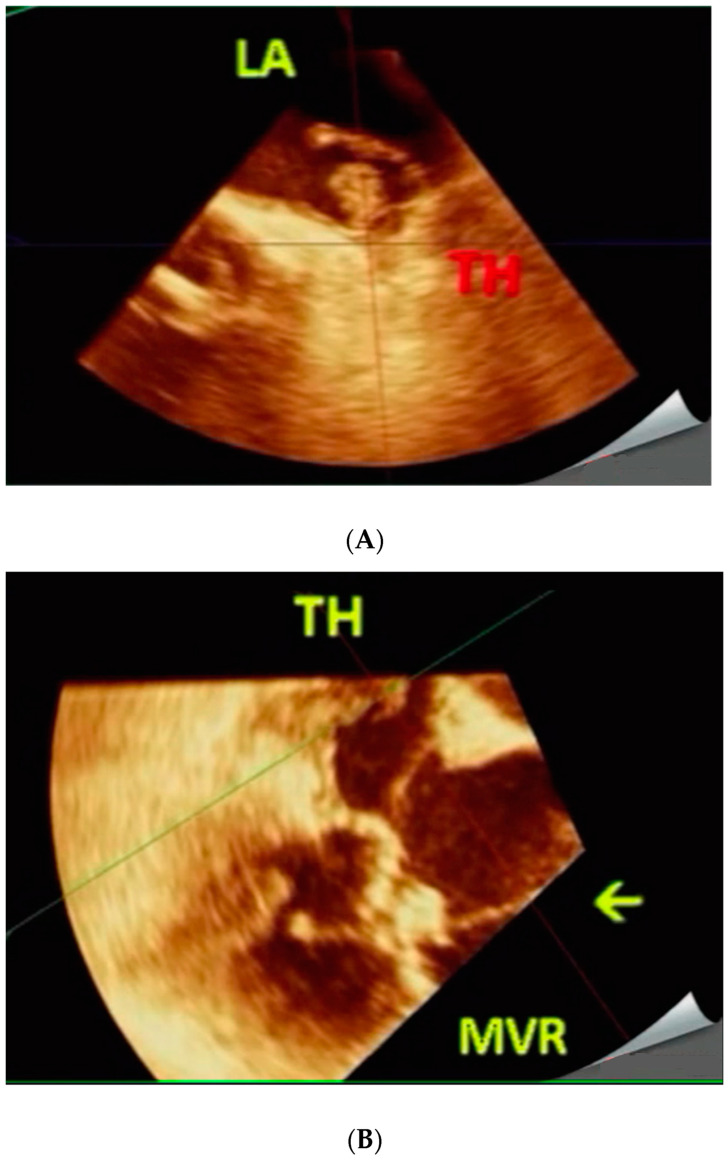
(A,B). 56-year-old female with severe MVR stenosis (Case 2). Pre-procedure. Live/real-time three-dimensional transesophageal echocardiography (A,B). A TH is noted in LAA and adjoining LA body in A. During procedure. The arrow in B points to the catheter deployment device passing through MVR avoiding the TH. Abbreviations as in Figure 1.
